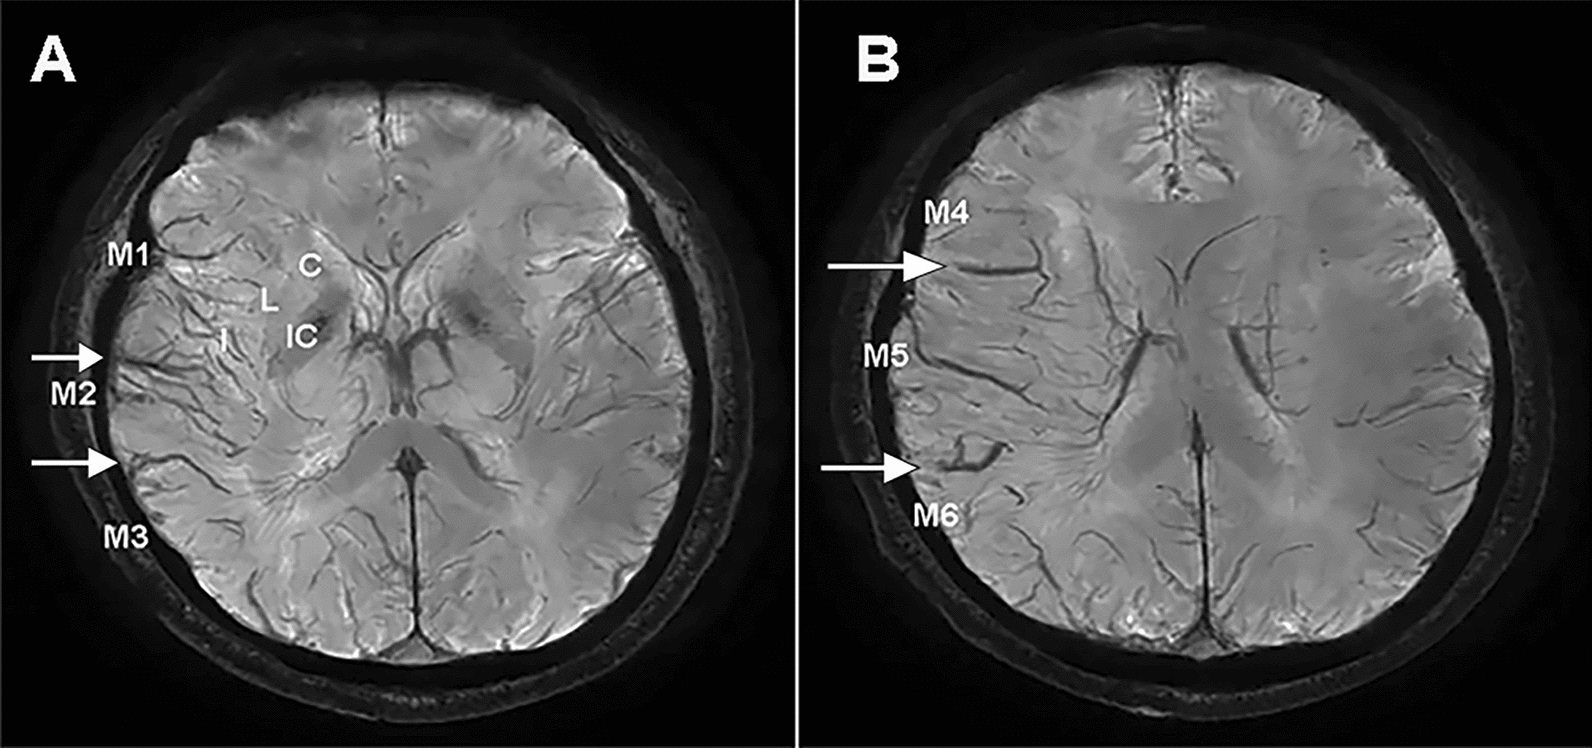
Example of APVS-ASPECTS score. A typical extensive APVS image which are visible on seven areas of the middle cerebral artery (MCA) territory (M1, M2, M3, Insula, M4, M5, M6) and the APVS-ASPECTS score was 7
